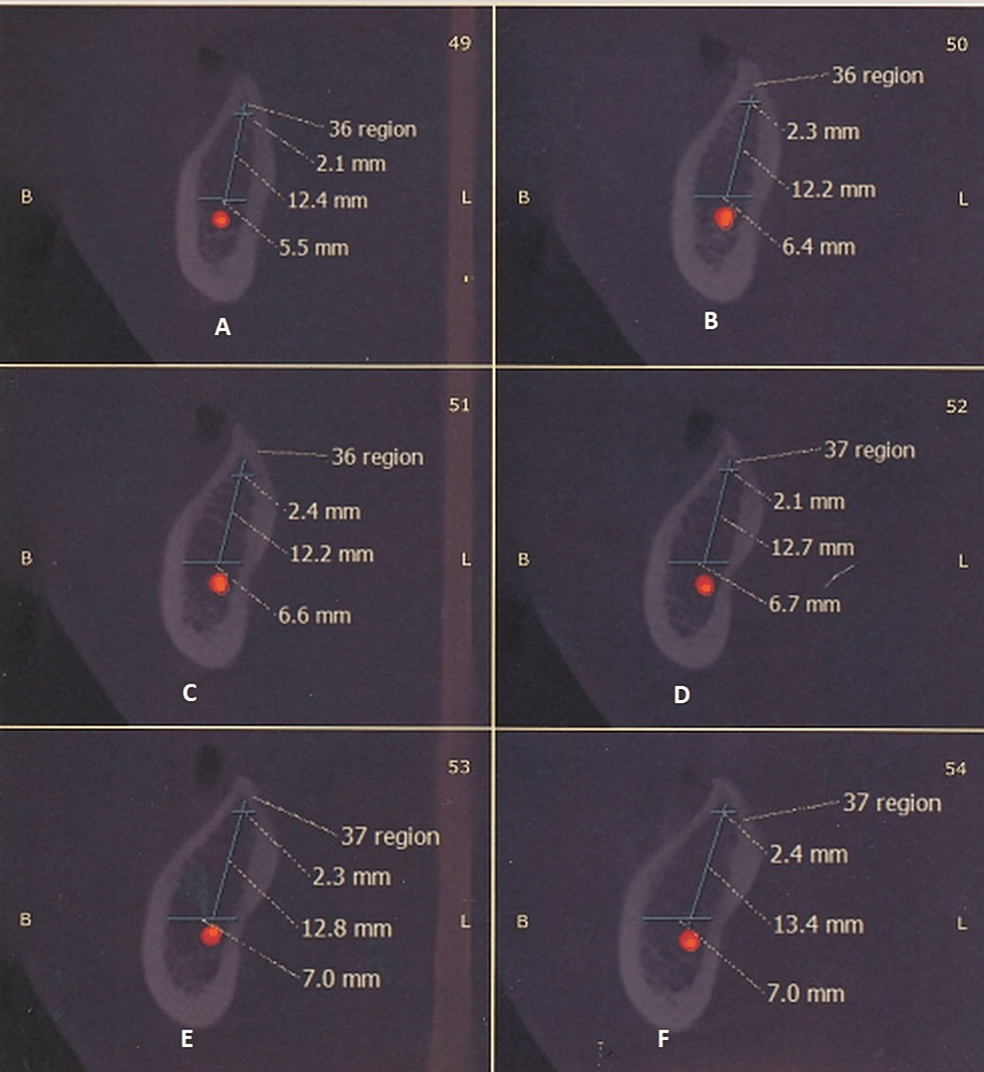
CBCT scans showing the measurements of bone ridge thickness A, B, and C show the 36 region, and D, E, and F show the 37 region CBCT: cone-beam computed tomography
